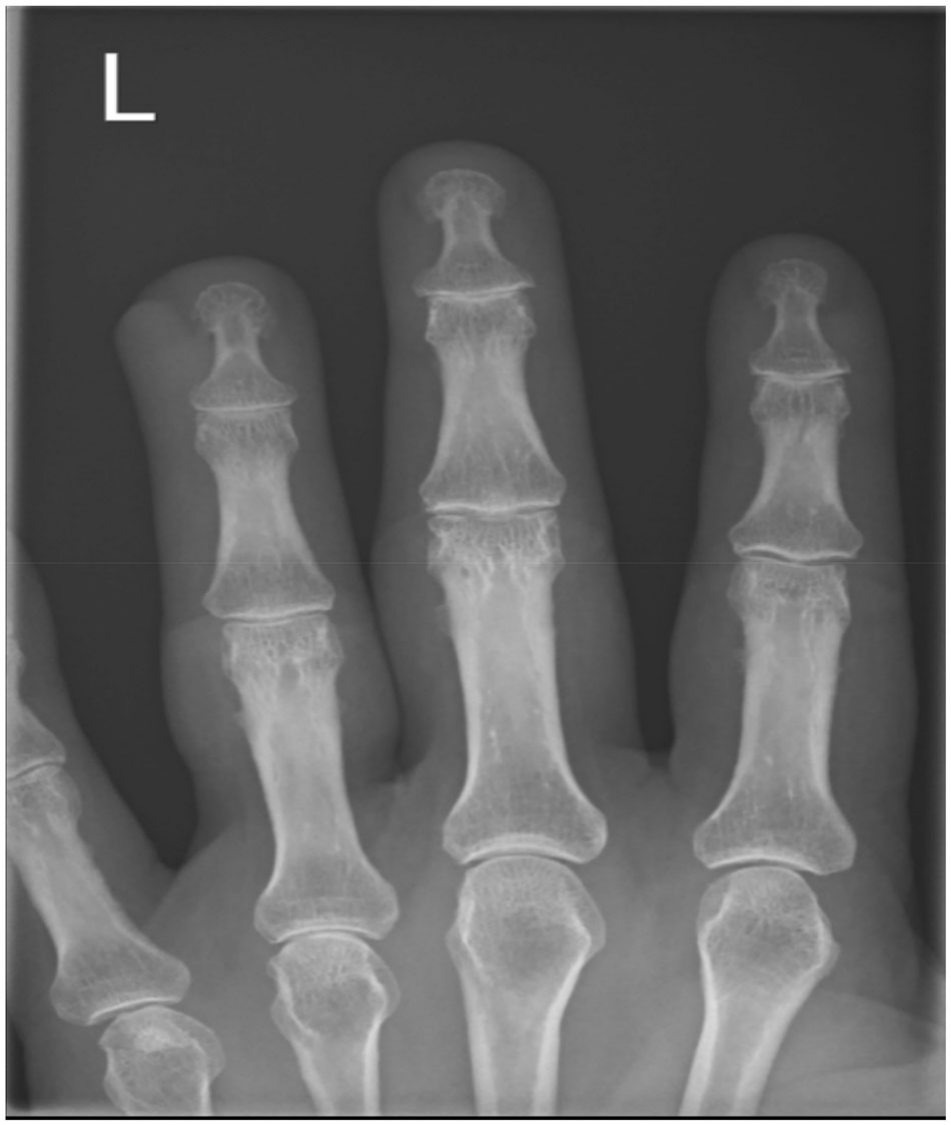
Radiographs of the left hand ring finger – PA view of the Left Index, Middle and Ring Fingers no obvious osseous pathology, left ring finger – increase density over the distal ulnar aspect of the left ring finger.
